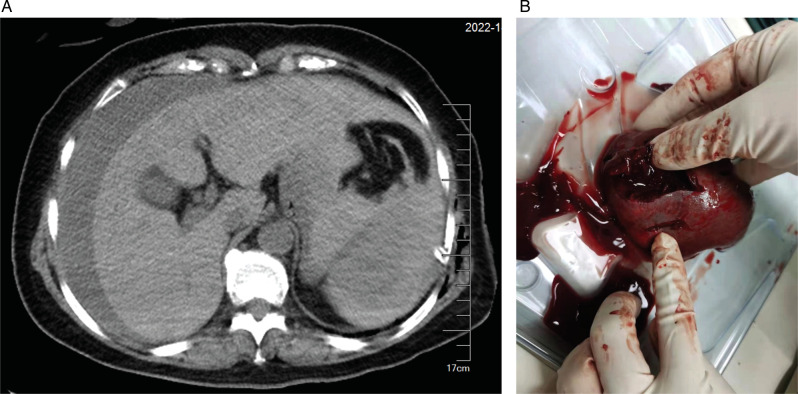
Images of abdominal computed tomography and ruptured spleen. (A) Abdominal computed tomography showing splenic hematoma and large amount of free intraperitoneal fluid. (B) Two lacerations of the ruptured spleen.
